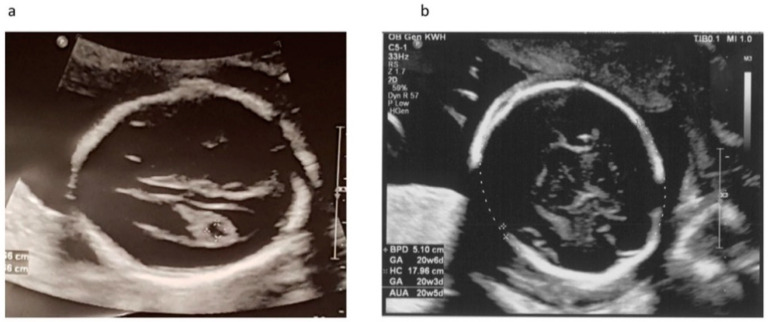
Ultrasound scan at 21 and 23 weeks of gestation. (a) Transventricular plane at 21 weeks showed unilateral choroid plexus cyst which was resolved later in scan at 23 weeks; (b) Biparietal diameter and head circumference at 23 weeks.
